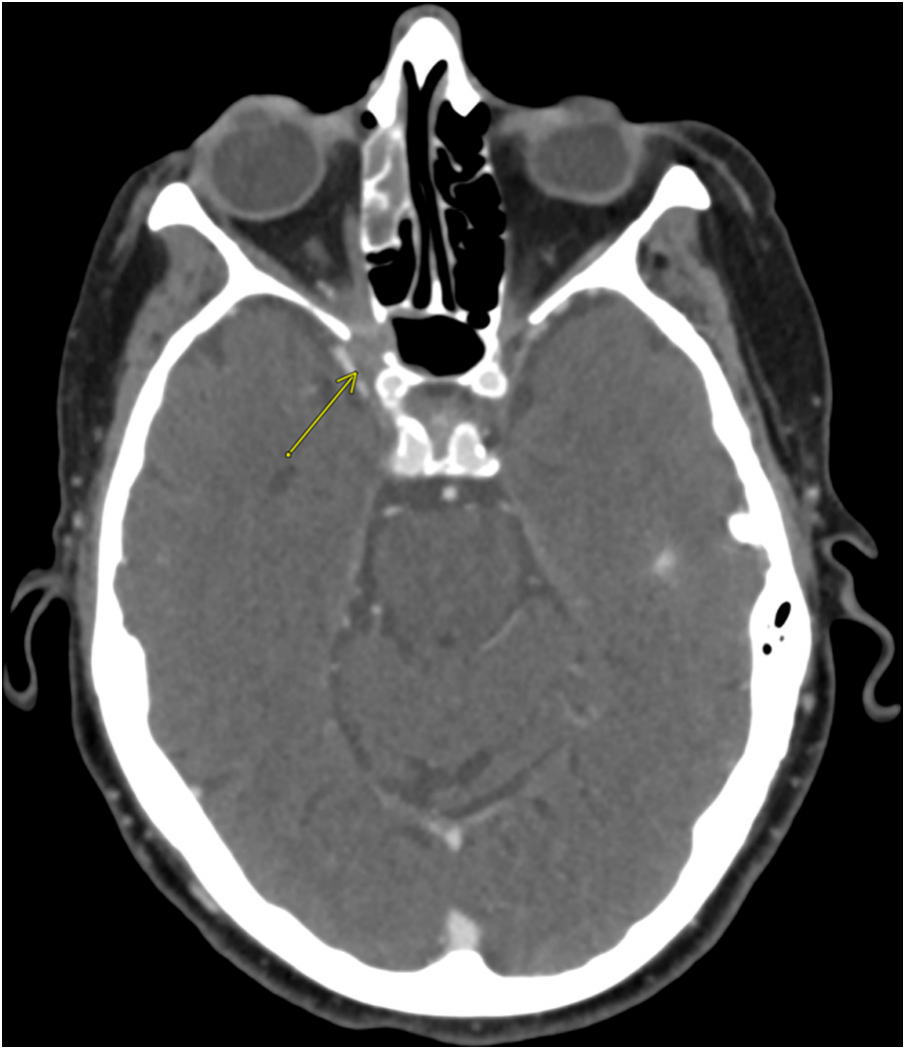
CT of the head and orbits with IV contrast demonstrating soft tissue thickening and indistinctness of fat in the region of the right optic canal and right orbital apex and an unusual bone defect in the right lateral wall of the right sphenoidal sinus.
